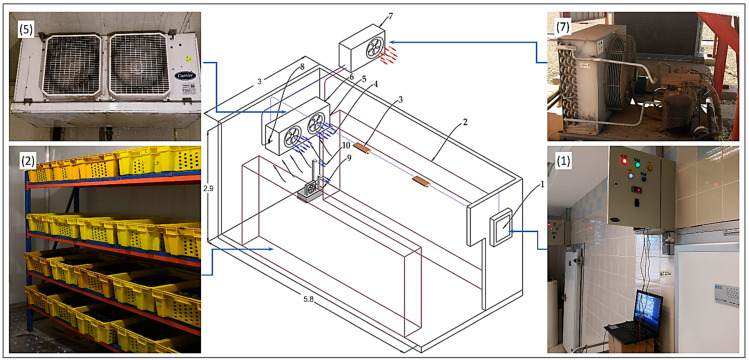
The main component of the cold storage room (dimensions in m). (1) Control unit, (2) storage shelves, (3) LED light bulbs, (4) cold air direction, (5) evaporator unit, (6) electrically operated expansion device, (7) condensing unit, (8) pressure transmitter, (9) ultrasonic humidifier, (10) outputted mist (all dimensions in meters).
